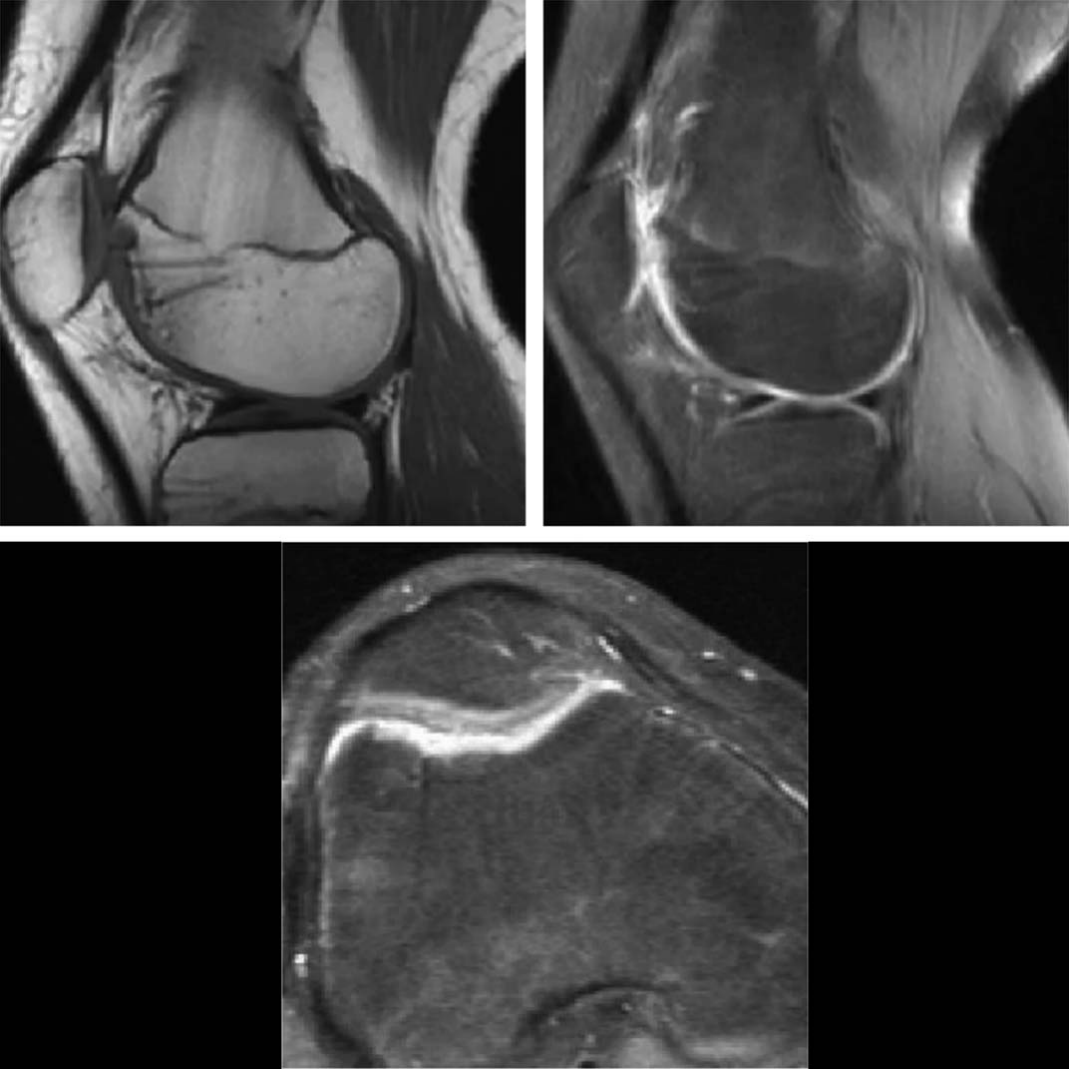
Knee MRI images 6 months postoperatively. Full consolidation of the osteochondral fragment in the T1- and T2-weighted images.
